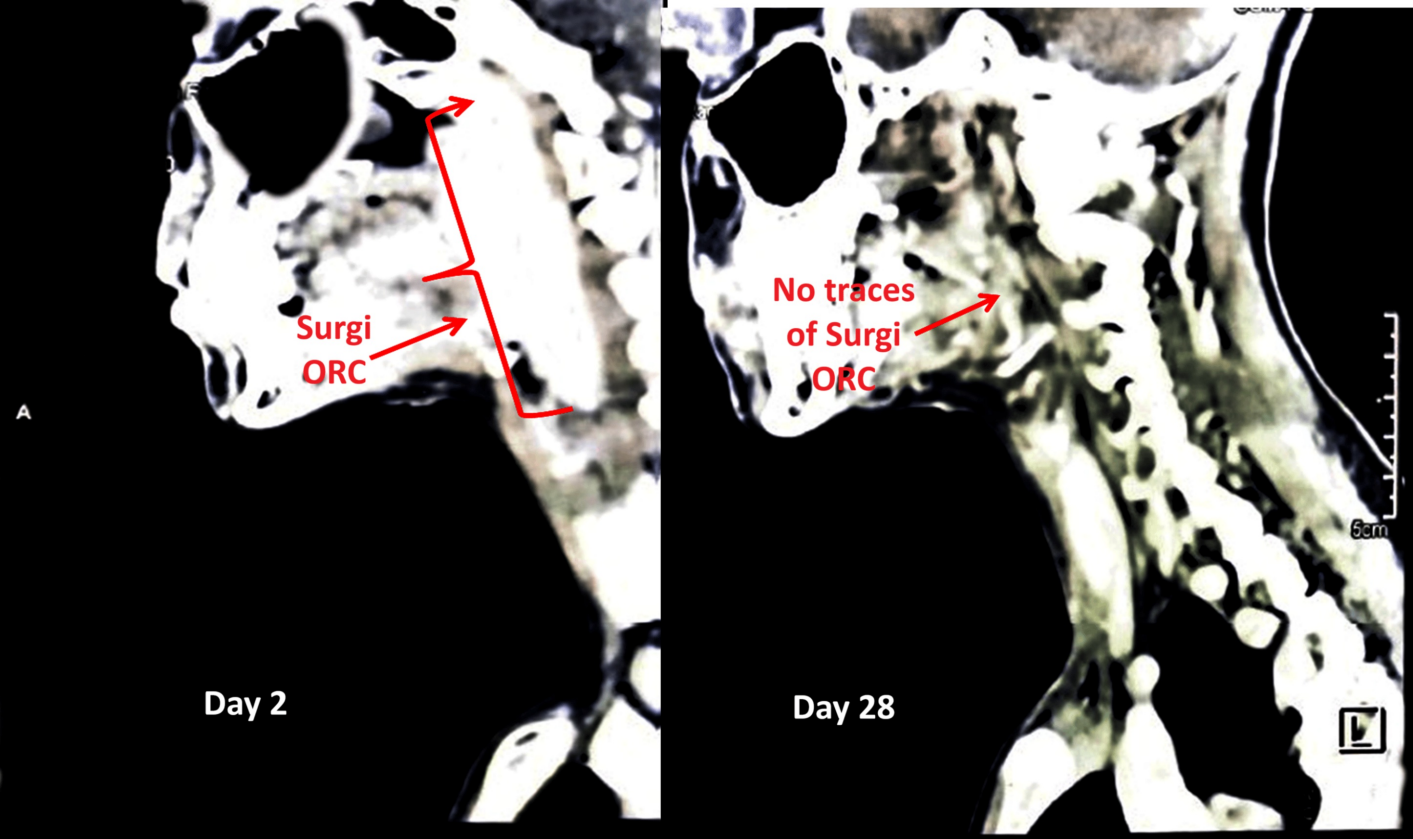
CT scan images of case 4 Day 2 (left panel): Surgi-ORC® is clearly visible in the operated region (arrows). Day 28 (right panel): no traces of Surgi-ORC® are observed, indicating complete resorption.
